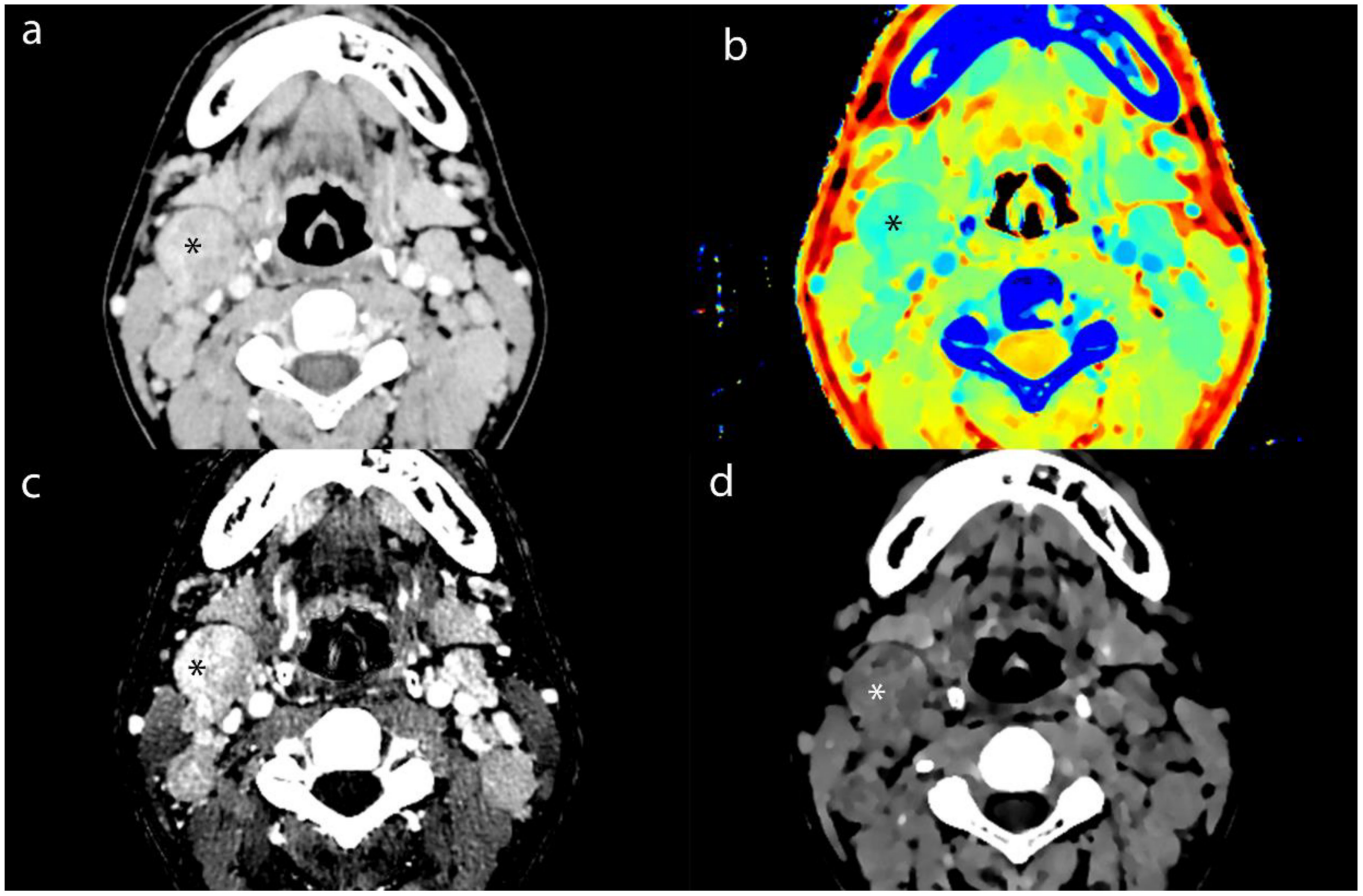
Axial contrast-enhanced CT images of MLN (*) from a 16-year-old male patient with NPC. (a) shows the short-axis diameter and CT value of the MLN. (b)- (d) shows the Zeff maps, Iodine no water maps and ED maps of MLN.
